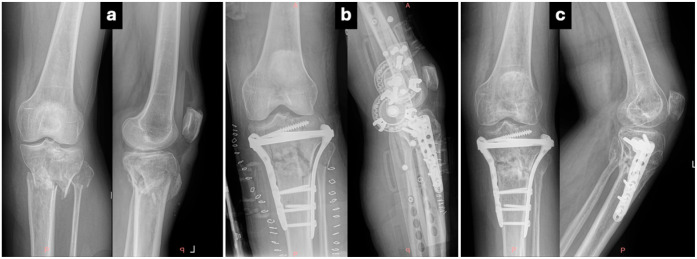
Preoperative X-rays of a 43-year-old female patient with a Schatzker VI tibial plateau fracture (a). Post-operative X-rays with both condyle plating (anterolateral and medial) after reduction via a dual approach (b). X-rays at 3-month follow-up reveal satisfying healing progress and preservation of plateau reduction despite slight anterior angulation in metaphysis, which increased the posterior slope of the tibial joint surface (c).
